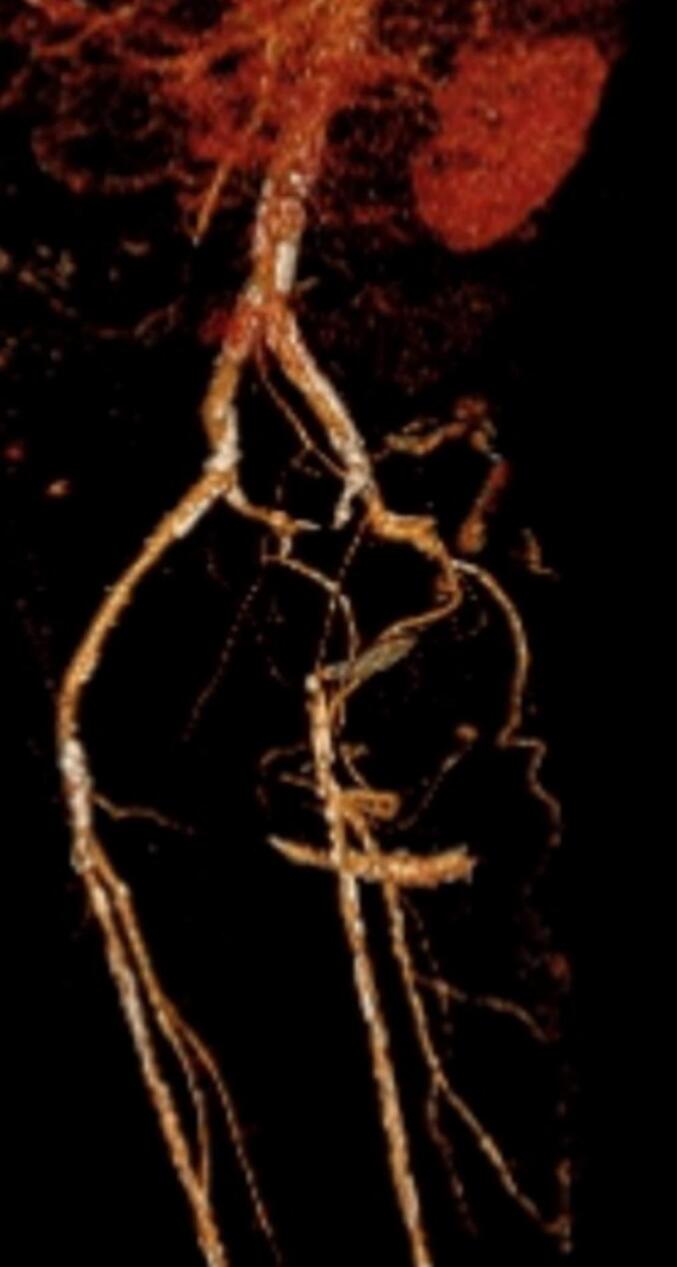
CT angiography showed a thrombo-embolic occlusion of the left external iliac artery and common femoral artery. However, the left superficial femoral artery and the left profunda femoris artery were visible from their origins.
